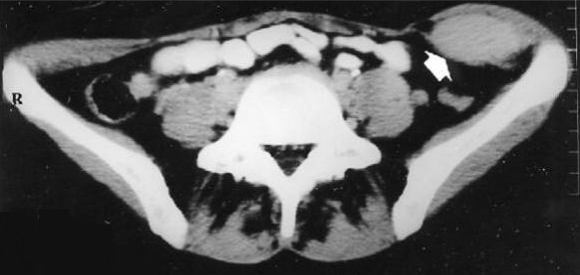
CT Scan of the patient demonstrating the tumour mass
